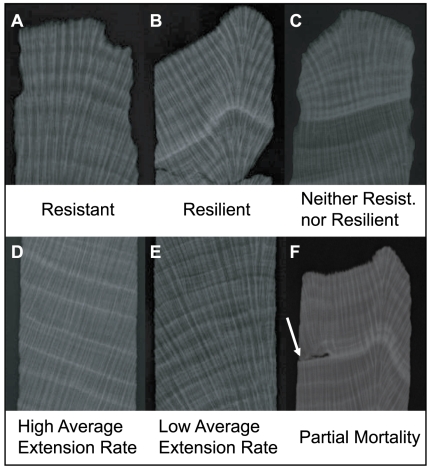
X-radiographs of various coral cores showing the different types of growth behavior discussed. (A) Coral without the 1998 growth suppression, indicating resistance to bleaching in 1998. (B) Coral with the 1998 growth suppression, recognized by the bright high-density band, but with a quick return to pre-1998 extension rates, indicating resilience after bleaching. (C) Coral with the 1998 growth suppression and continuing depressed extension rates after 1998, indicating a lack of both resistance and resilience to bleaching. (D) A coral with relatively high average extension rate. (E) A coral with relatively low average extension rate. (F) A coral with a partial mortality scar on the left (noted by white arrow), coincident with the 1998 growth anomaly.
